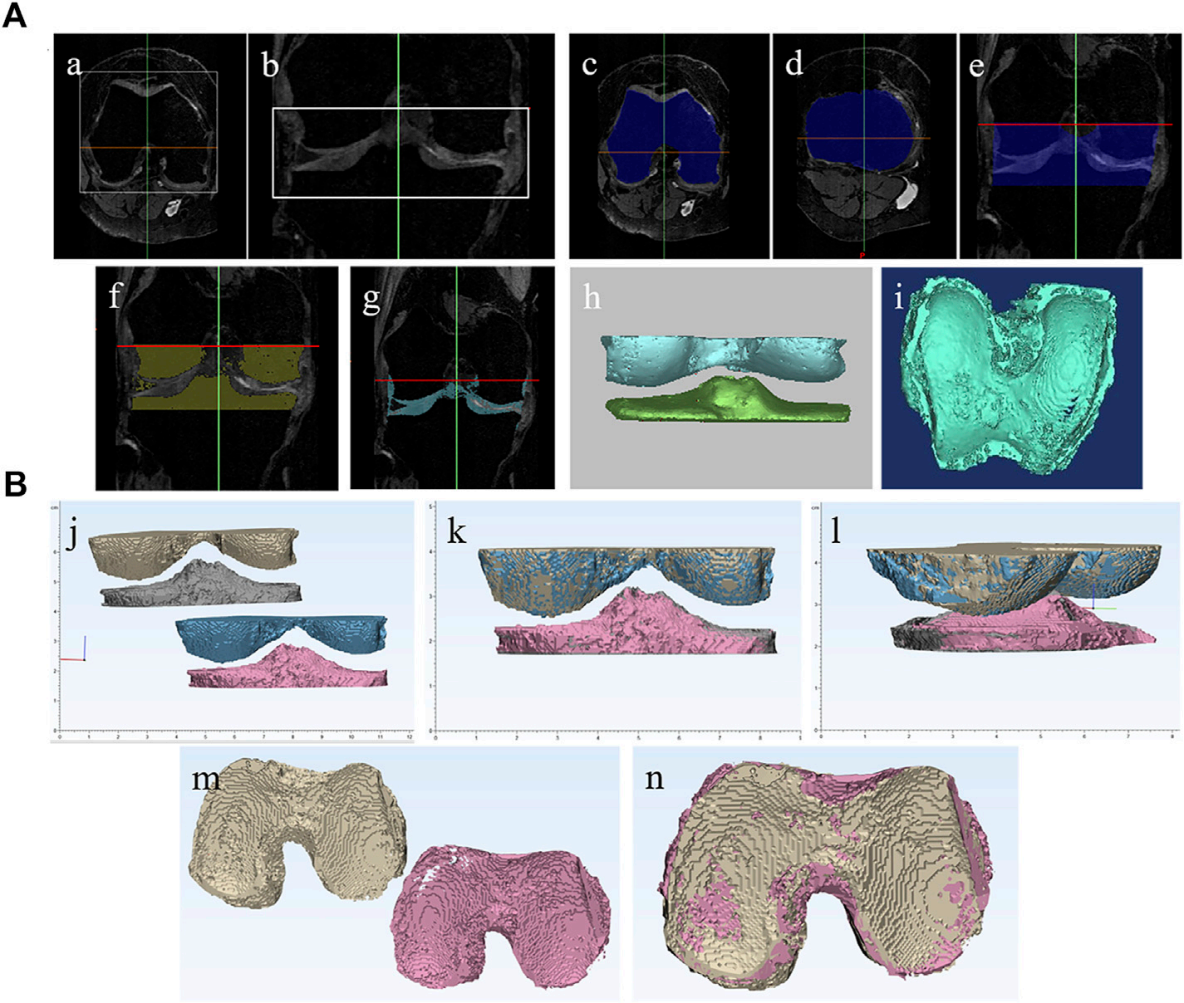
Establishment of human knee joint MRI 3D models and 3D spatial alignment method. (A) Establishment of human knee joint MRI 3D models: (a,b) The detection range from the femoral condyle to the tibial plateau of knee joint is selected in coronal plane and sagittal plane of MRI; (c–e) region growing method is used to obtain the contour curves of all planes of the detection range; (f,g) Based on the gray scale thresholds of cartilage or bone, the scanning sequences of cartilage or bone within the selected region are identified respectively. (h,i) the cartilage and bone 3D MRI models are generated. (B). Establishment of 3D spatial alignment method: (j-n) 3-matic was applied to spatially align the MRI image data I and II based on osteogenic section to ensure that the cartilage section is registered in the same 3D spatial domain.
